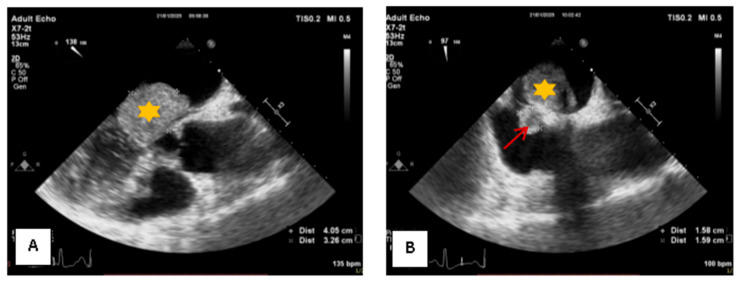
Transesophageal Echocardiography: (A) The left atrial tumor, measuring 4.0 × 3.2 cm, is marked with a yellow asterisk. (B) The right atrial tumor, measuring 1.5 × 1.5 cm, is marked with a red arrow.
